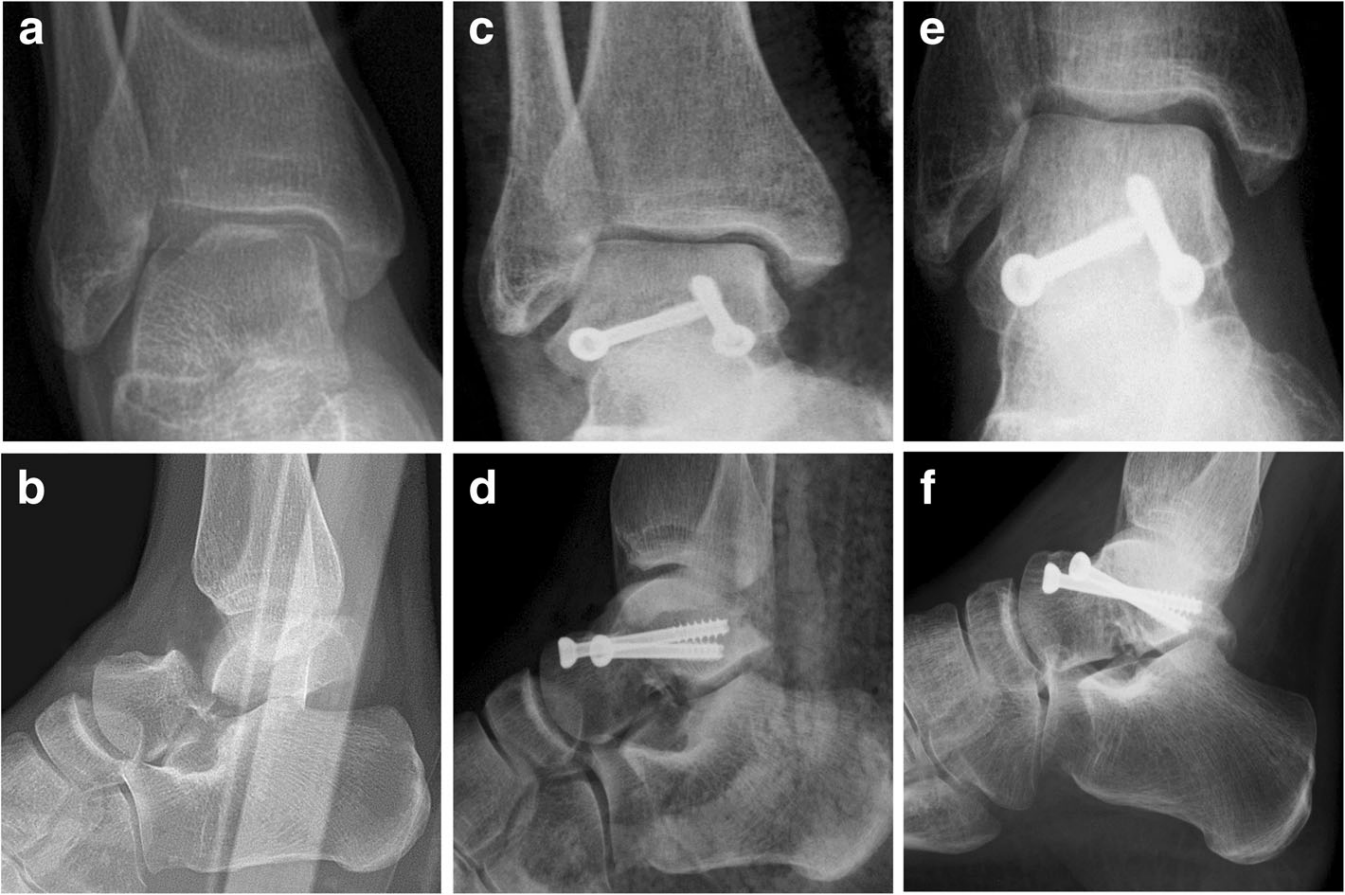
Case 1: a 40-year-old woman with a Hawkins type III fracture presenting signs of PTA and AVN, but without collapse of talar dome at last follow-up. Antero-posterior and lateral radiographic images at preoperative period (a-b), immediate postoperative period (c-d), 88-month follow-up (e-f)
